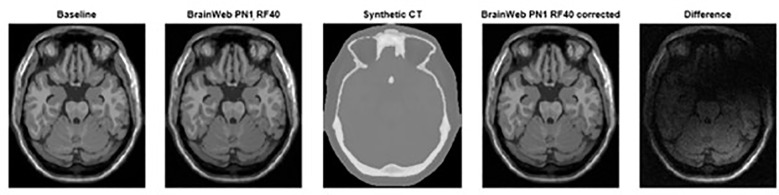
From left to Right, baseline MRI (PN0%, RF0%), distorted MRI (PN1%, RF40%), synthetic CT, and corrected distorted MRI (PN1%, RF40% - based on sCT correction) datasets including the difference observed using image subtraction between corrected and non-corrected datasets.
